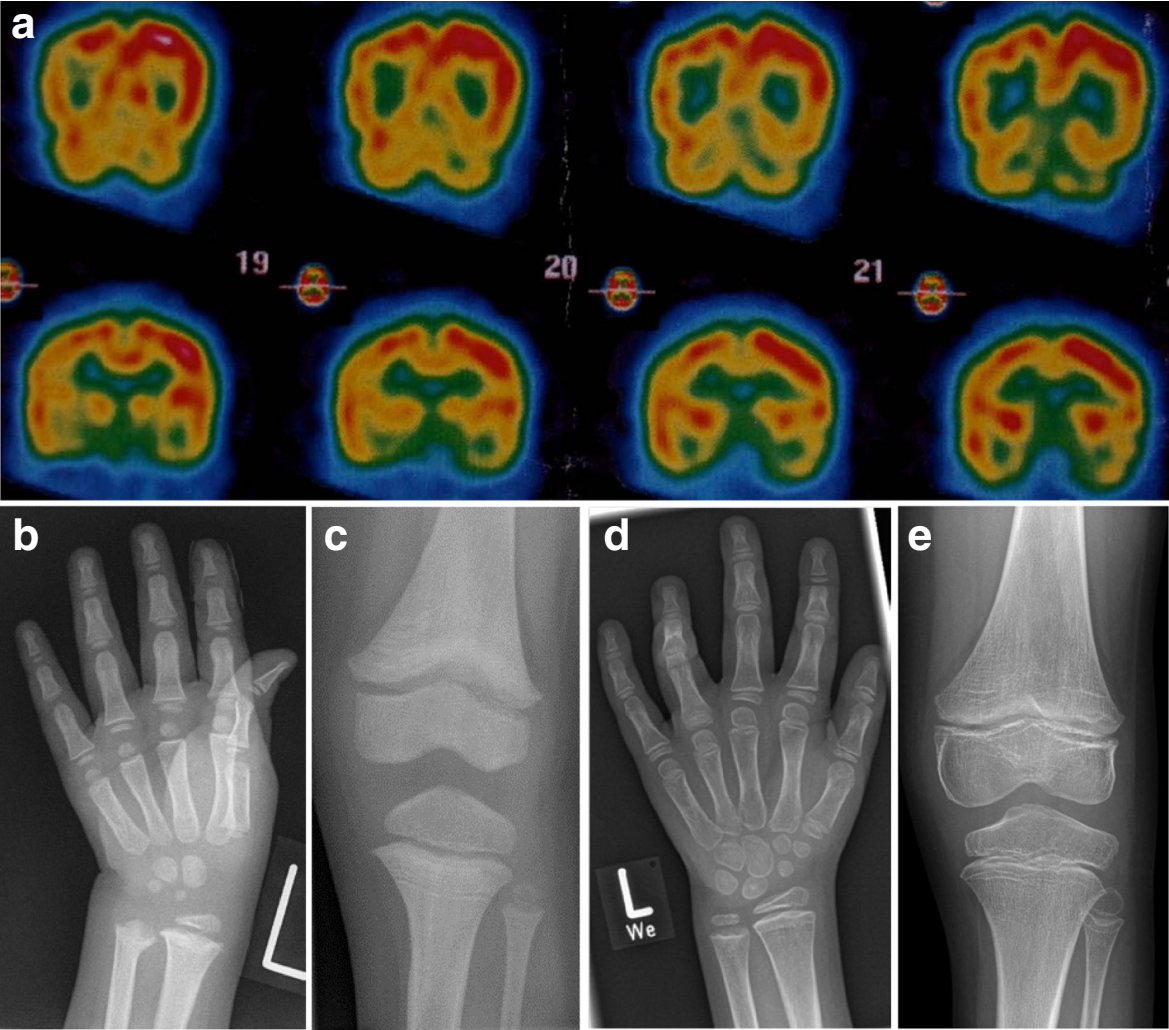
Clinical findings. a Single-photon emission computed tomography (SPECT) of the brain at 5 years of age demonstrating speckled areas of reduced perfusion predominantly in the frontal, temporal and parietal regions of the right cortex as well as in parts of the basal ganglia consistent with cerebral vascular lesions. Radiographs of the left hand and knee taken at 4 (b, c) and 9 years of age (d, e), respectively, showing discrete metaphyseal irregularities. In addition, reduced mineralization of the lower extremities caused by impaired physical activity is seen at 9 years of age
